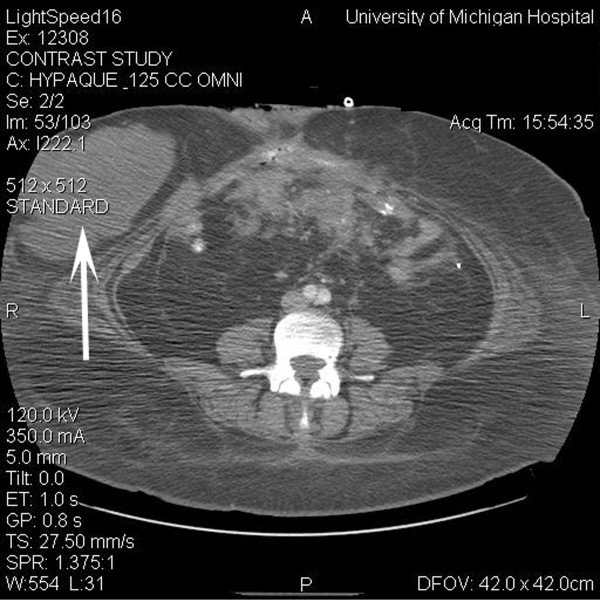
Computed tomogram of the abdomen at the same level as Figure 2 following cytotoxic chemotherapy. The largest abdominal wall lesion decreased to 13 × 7 cm (arrow). Other lesions in the rectus sheath decreased from 6.4 × 3.4 cm and 4.4 × 2.7 cm to 6 × 3 cm and 3.3 × 2.2 cm, respectively. The mesenteric masses decreased markedly.
